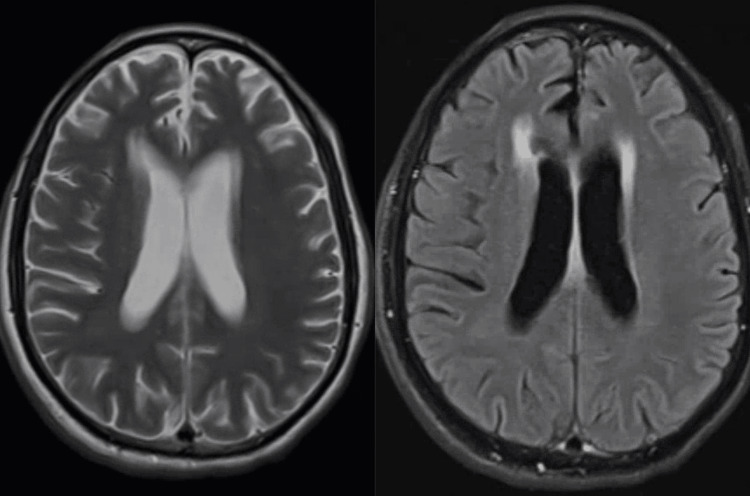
Axial T2/FLAIR image above demonstrates a small, ill-defined hyperintensity in the right posterior frontal subcortical white matter, consistent with gliosis related to chronic microvascular disease. FLAIR: fluid-attenuated inversion recovery
